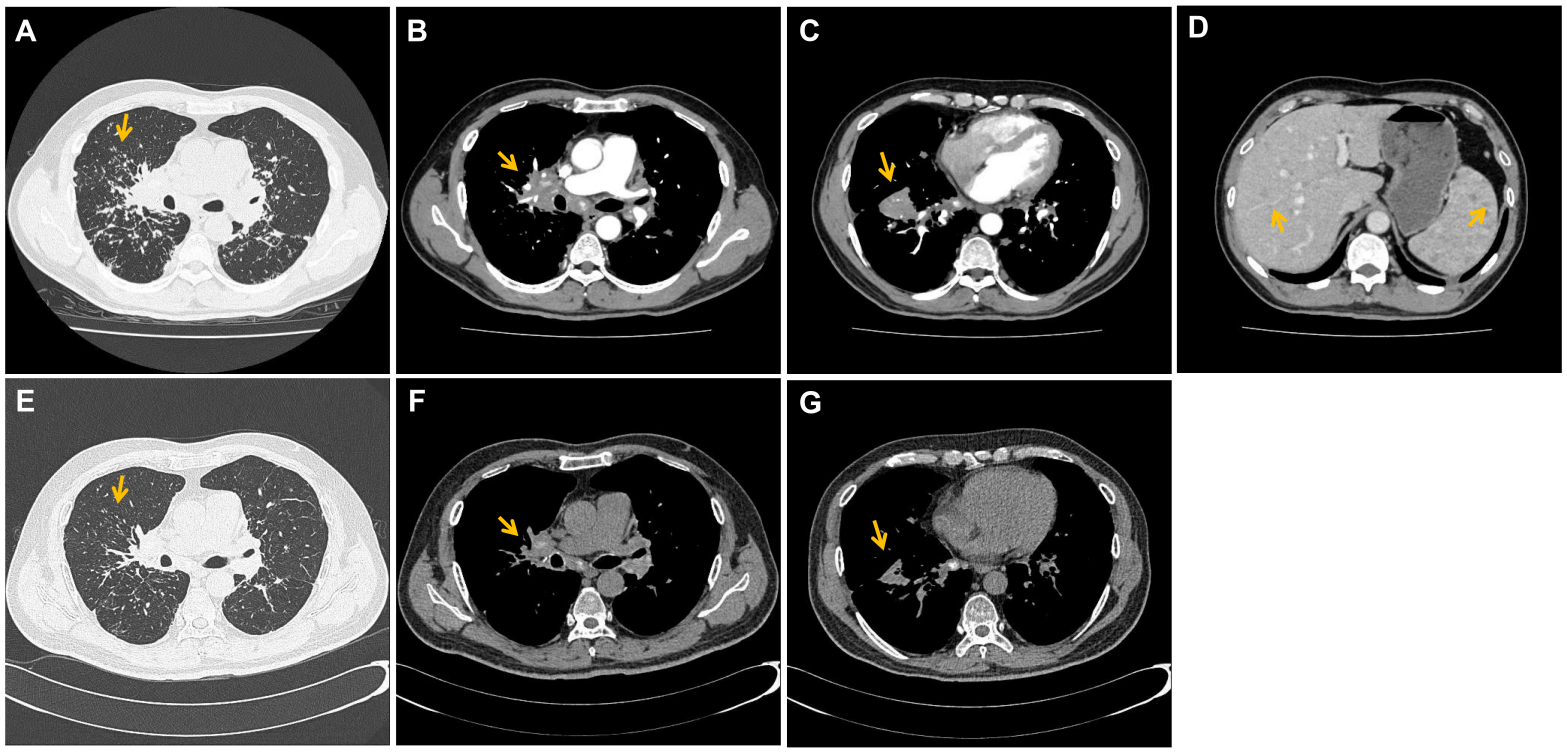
The initial and follow-up CT images of patient. (A) Computed tomography (CT) of the chest displayed diffuse nodules and consolidation in the lungs (yellow arrow). (B) Additionally, enlarged axillary and paraspinal lymph nodes with calcification and mild degree of enhancement were found in the bilateral mediastinum (yellow arrows); (C) as well as a mildly enhancement mass (about 5.5 × 3.4 cm) where a CT-guided percutaneous lung biopsy was performed (yellow arrows); (D) diffuse nodules in the liver and spleen were also detected (yellow arrows); (E–G) were after 2 months treatment. The condition of nodules, consolidation, lymphadenopathy and the mass showed significant improvement (yellow arrow).
